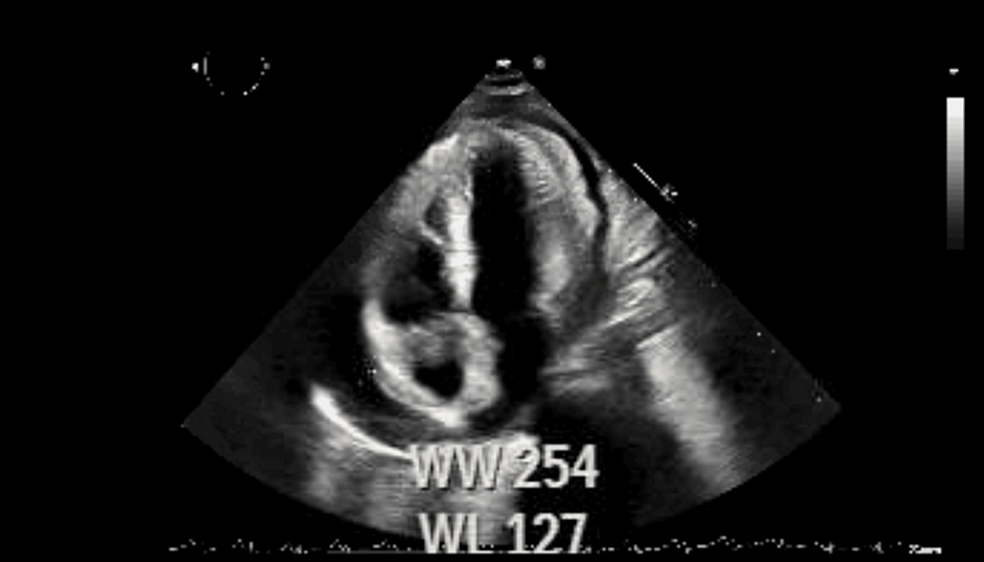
The early signs of cardiac tamponade of right-sided heart collapse are shown in the echocardiogram.
